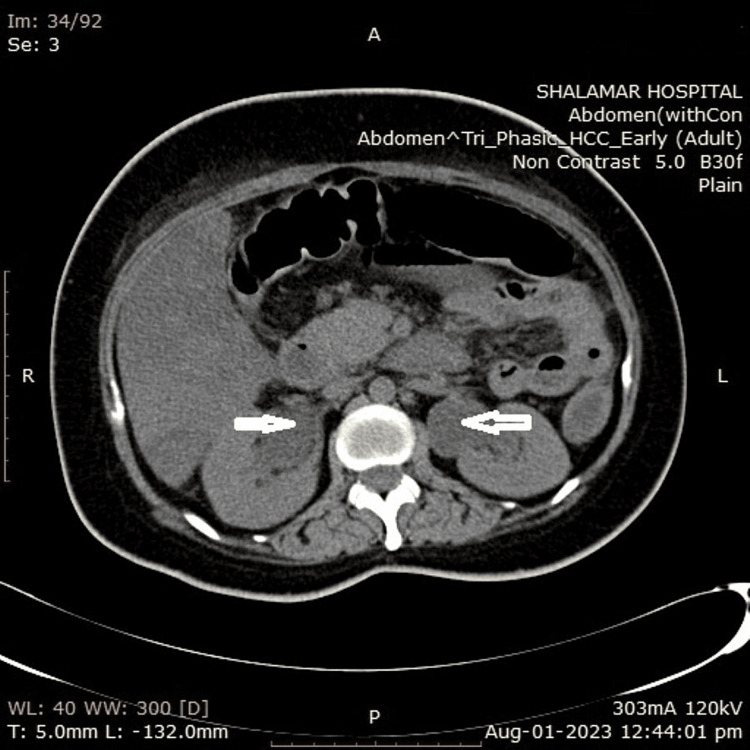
CT of the abdomen showing bilateral hydronephroureter (white arrows).
